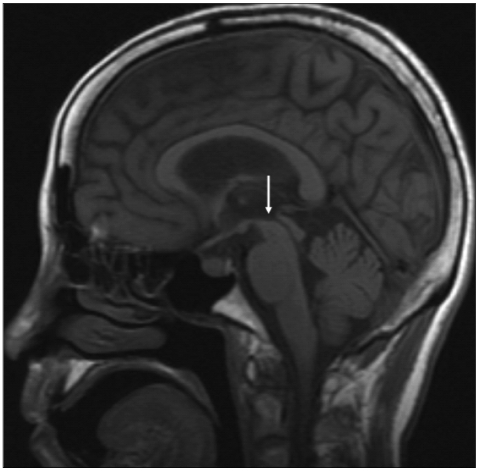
Magnetic resonance imaging. The midsagittal image shows marked atrophy of the midbrain tegmentum and the "penguin silhouette" sign (arrow). The midbrain area was 65 mm^2^ and the pons area was 550 mm^2^. Therefore, the ratio of the midbrain area to the pons area was less than 0.12.
